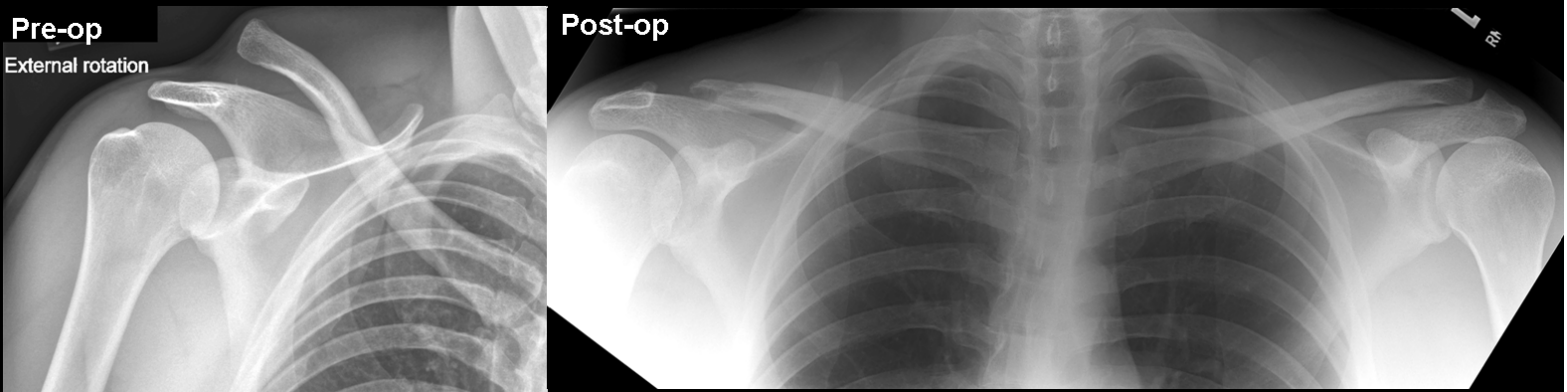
Preoperative and postoperative X-rays. Reprinted with permission: *OPERATIVE TECHNIQUES IN SPORTS MEDICINE, V12(1): 35–42, Millett PJ et al: "Acromioclavicular Joint Instability...". ^© ^2004 Elsevier Inc*
